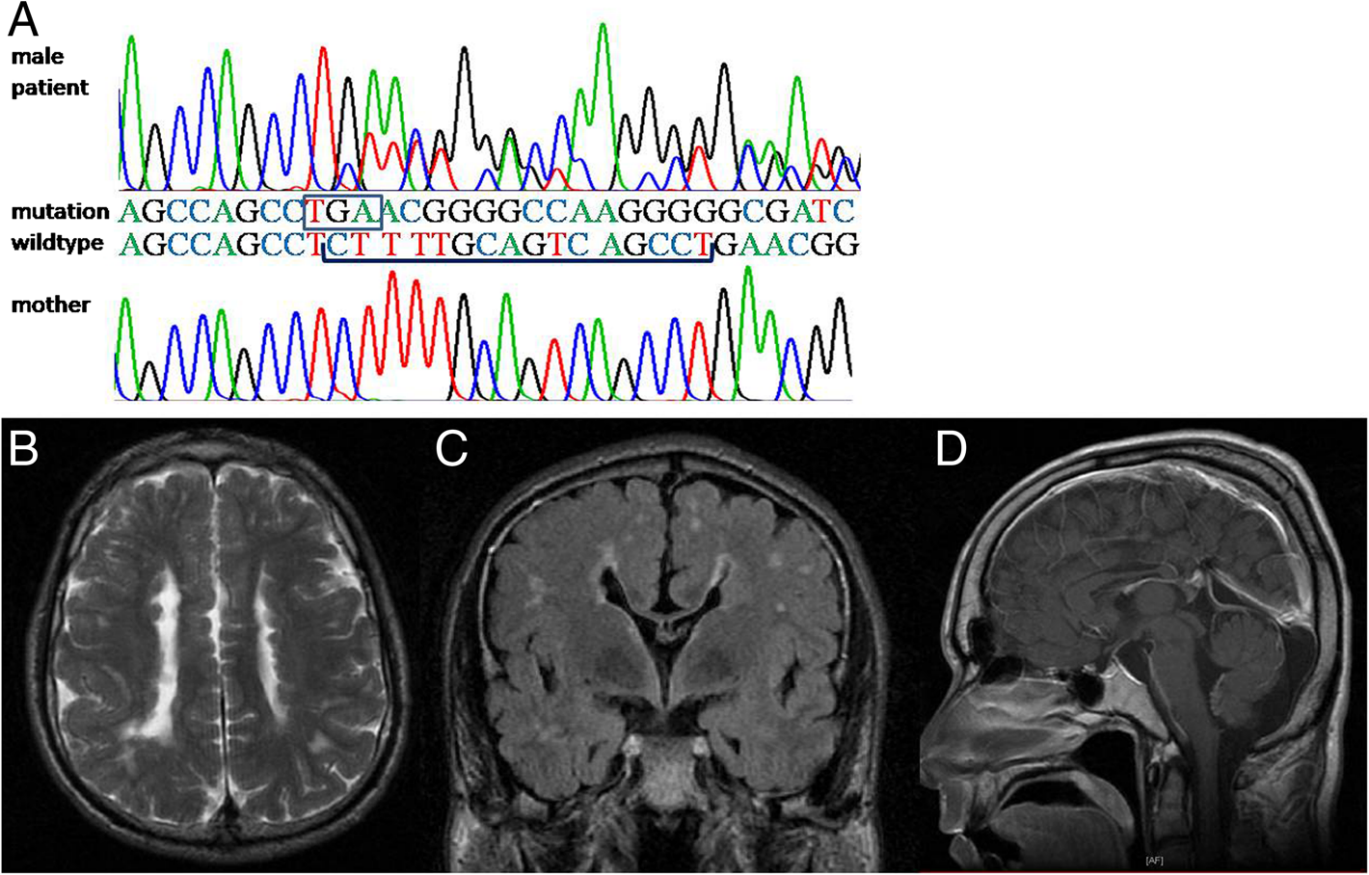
a High grade mosaic *FLNA* mutation c.7055_7070delCTTTTGCAGTCAGCCT in peripheral blood (*upper sequence*) of a 36 year old male patient 29 with normal male karyotype 46,XY, resulting in the nonsense mutation p.Ser2352*, novel stop codon boxed. Wildtype sequence of the healthy mother below. b–d cerebral MR imaging demonstrating extended PVNH bilaterally (b and c), diffuse white matter abnormalities and inward rotated anterior ventricular horns (c), hypoplastic corpus callosum and large mega cisterna magna (d)
